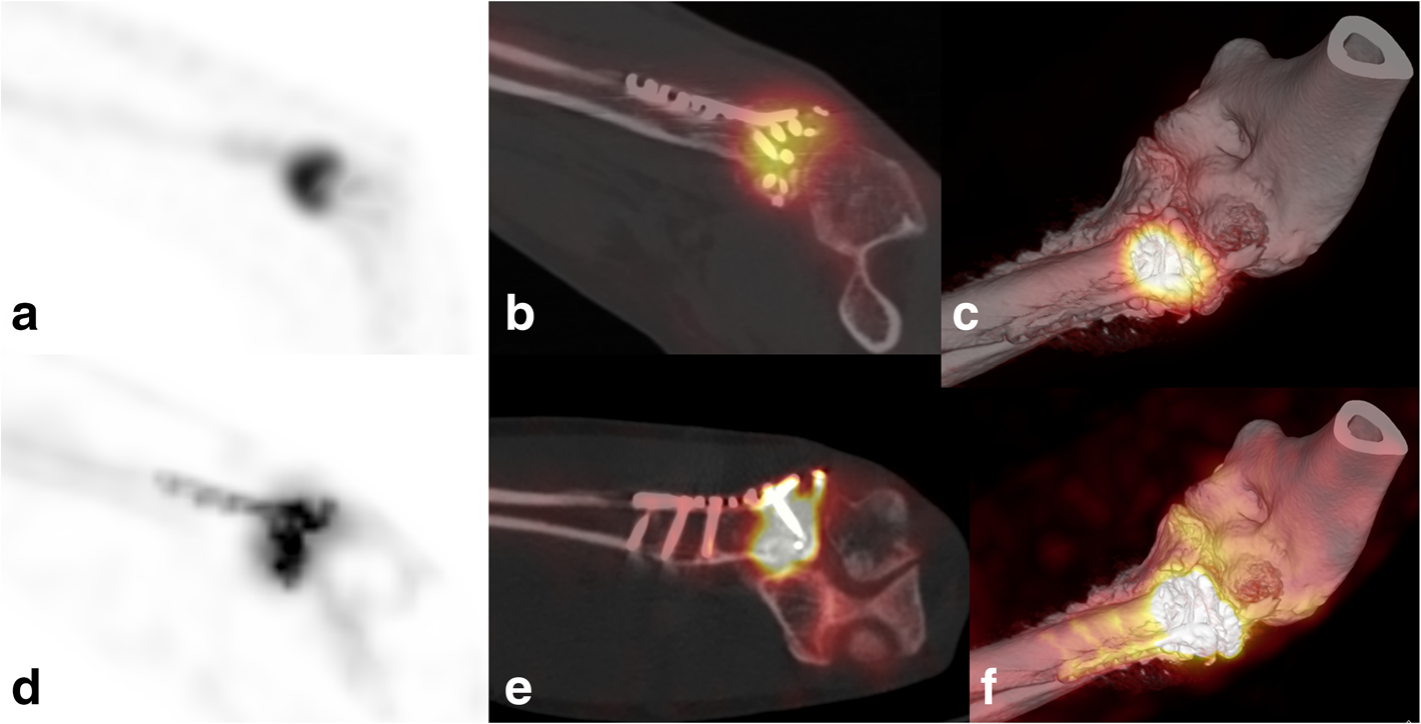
xSPECT Bone “shining metal artefact” in the elbow. SPECT/CT images of a 45-year-old male patient 6 months after osteosynthesis of a fracture of the proximal radius with persisting pain and suspicion for non-union. MIP image with xSPECT Bone reconstruction (d), fused sagittal SPECT/CT (e) and fused 3D reconstruction (f) show high “pseudouptake” of the plate and mini screws and true increased uptake in the non-union. Corresponding images (a–c) with conventional Flash3D reconstruction show no abnormal uptake of the radiotracer around the screws and no morphologic signs of loosening with maintained true uptake of the non-union. As a consequence of SPECT/CT, arthroplasty of the radial head was recommended
